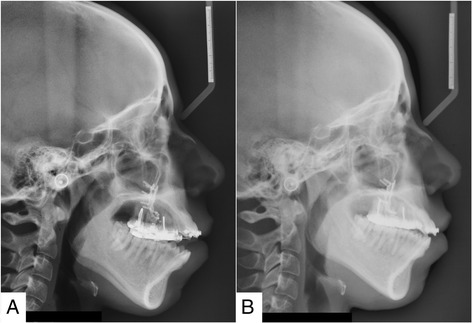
Lateral cephalometric radiographs of a subject: a before and b after maxillary posterior teeth intrusion
